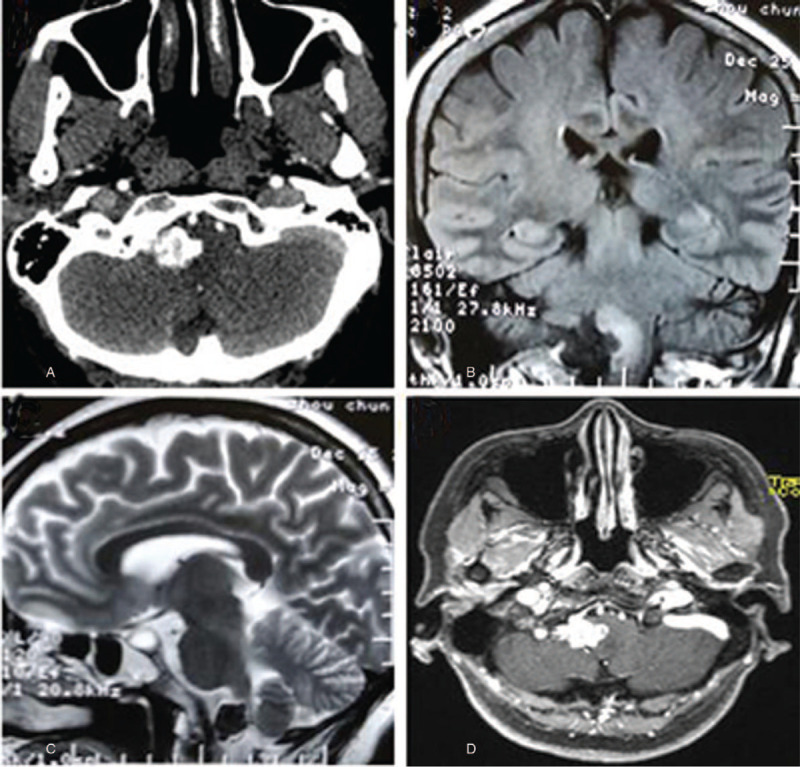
(A) Head computed tomography scan showing a lesion with a hyperattenuated signal in the right jugular foramen area. (B) Coronary T1 and (C) sagittal T2 MRI showing a hypointense lesion with edema of the adjacent brainstem parenchyma. (D) The lesion was homogenously enhanced after gadolinium administration.
